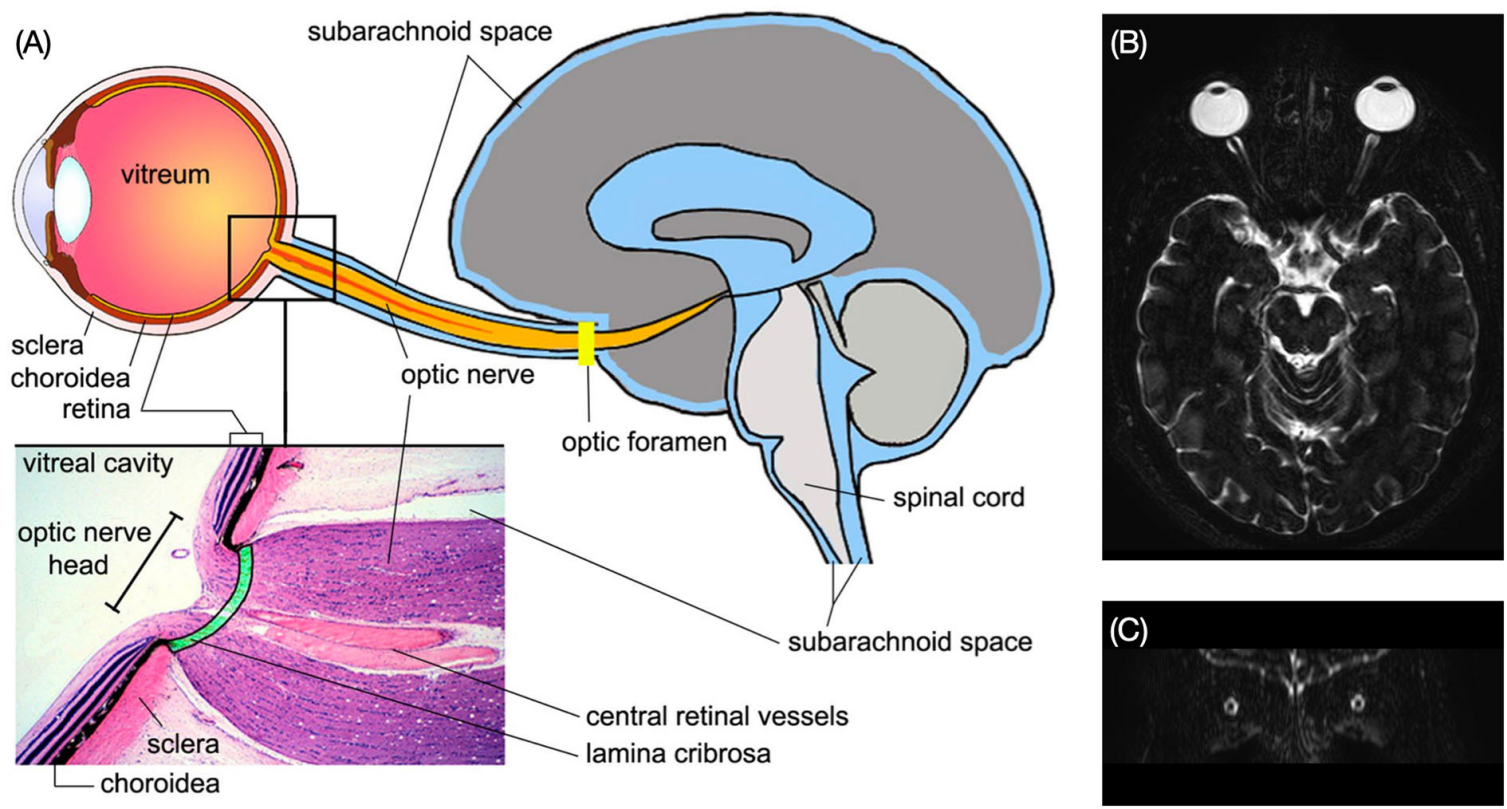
(A) Structure of the subarachnoid spaces of the ON and the brain. Enlarged view shows the structure of the ON head [Reproduced from Wostyn et al. (29) with permission]. (B) Axial MRI image of the brain shows the ON subarachnoid space in bright signal. (C) The ON subarachnoid space in coronal plane (Credit: Quan V. Hoang).
